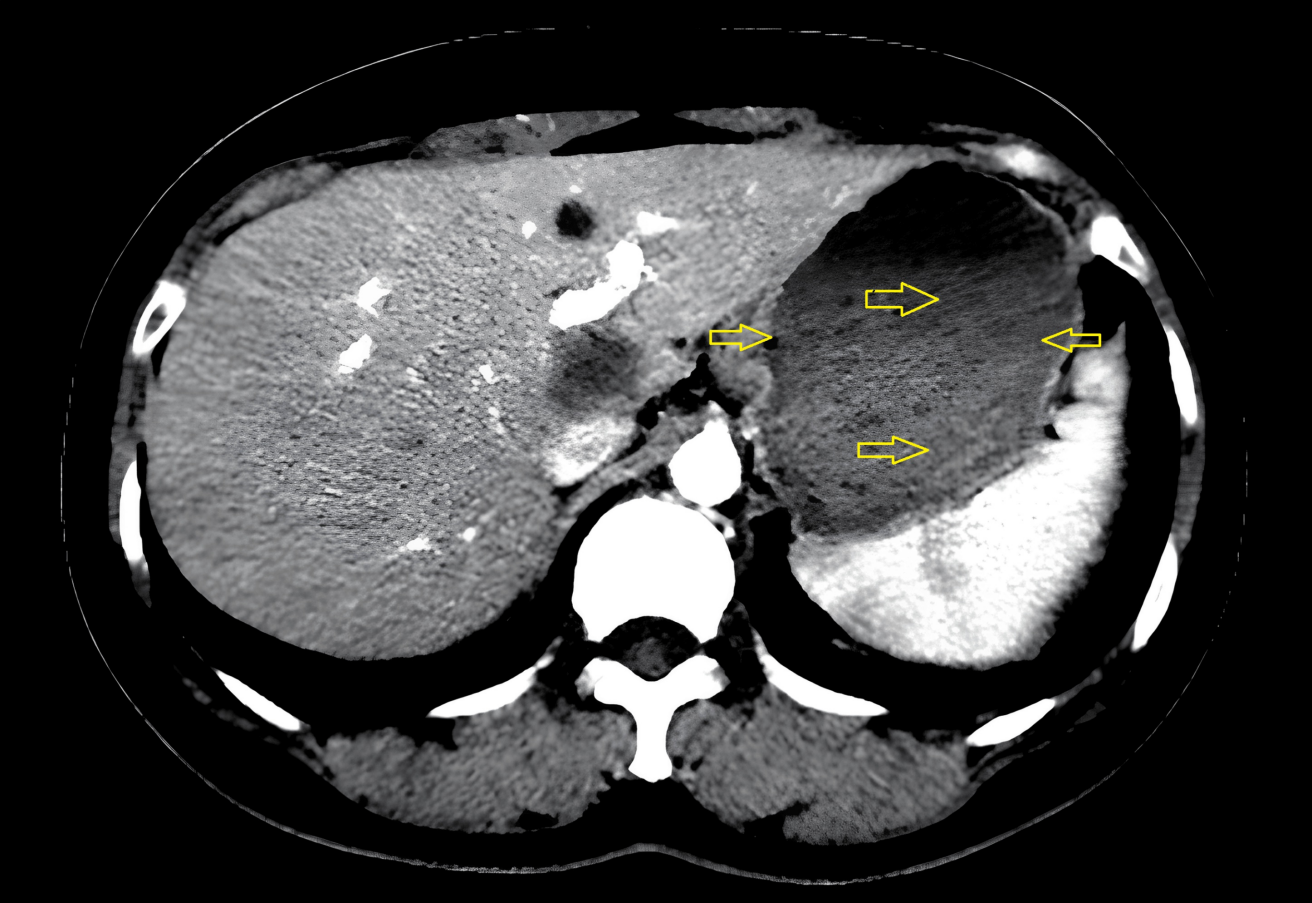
MRI of the liver revealing tumors measuring 50-54 mm in hepatic lobule VII.
